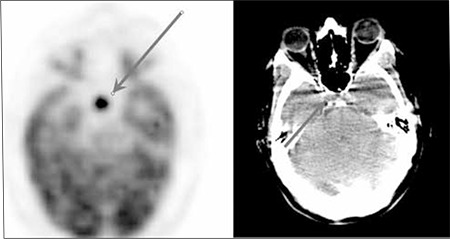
FDG PET and CT axial image : There is a focus of intense hypermetabolismin the sellar region which might be due to involvement ofpituitary gland with lymphoma or a benign pituitary adenoma
